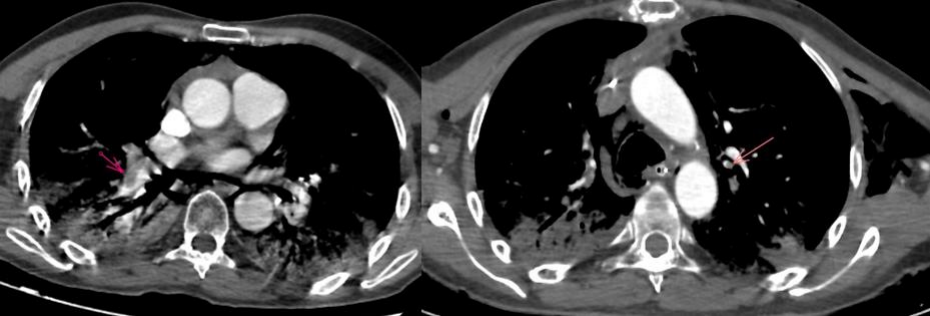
computed tomography pulmonary angiography in axial lung window showing acute bilateral pulmonary embolism (red arrows)
